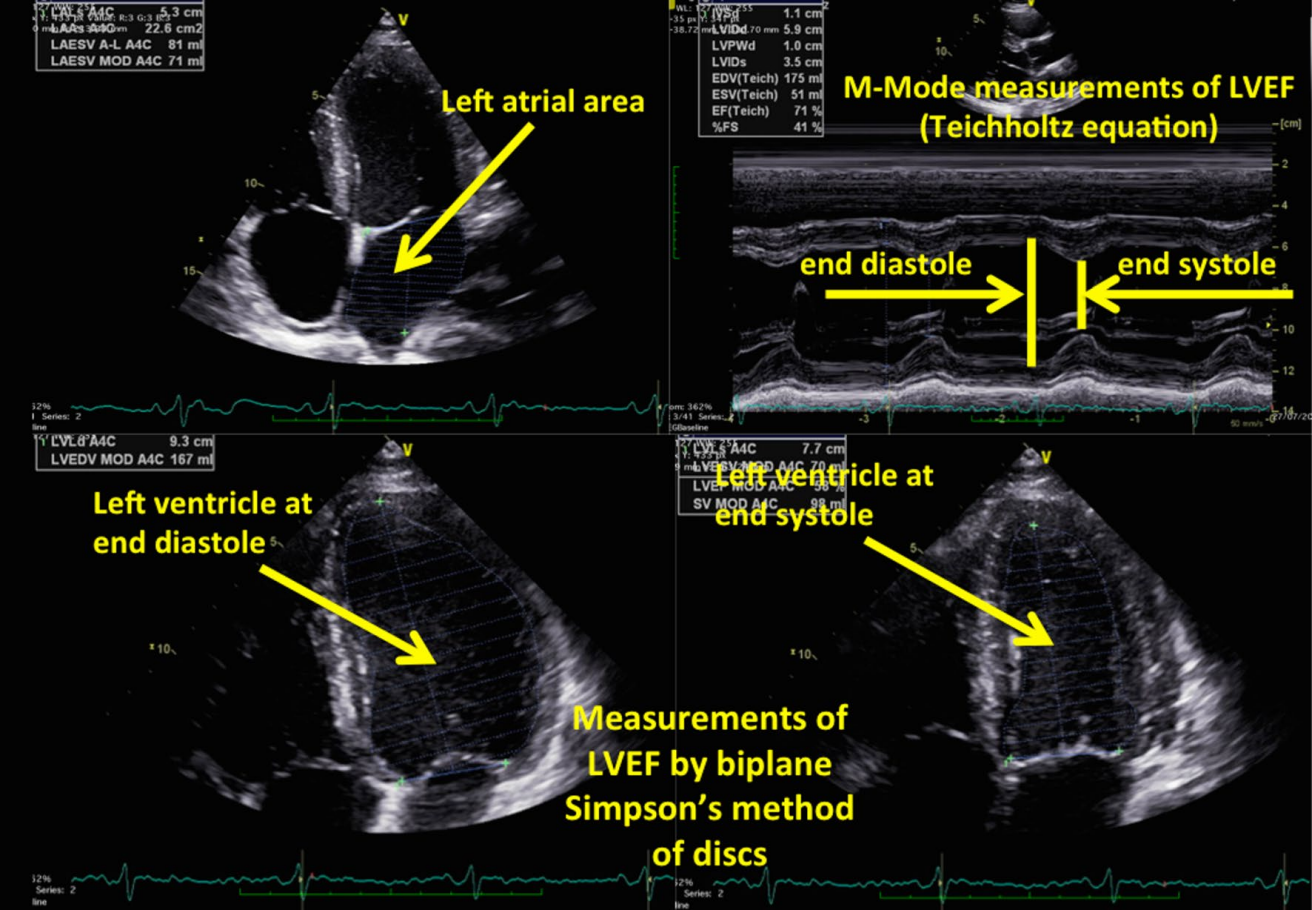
Echocardiographic assessment of left ventricular systolic function (Simpson’s method and Techoltz equation) as defined by Lang et al. [22]
